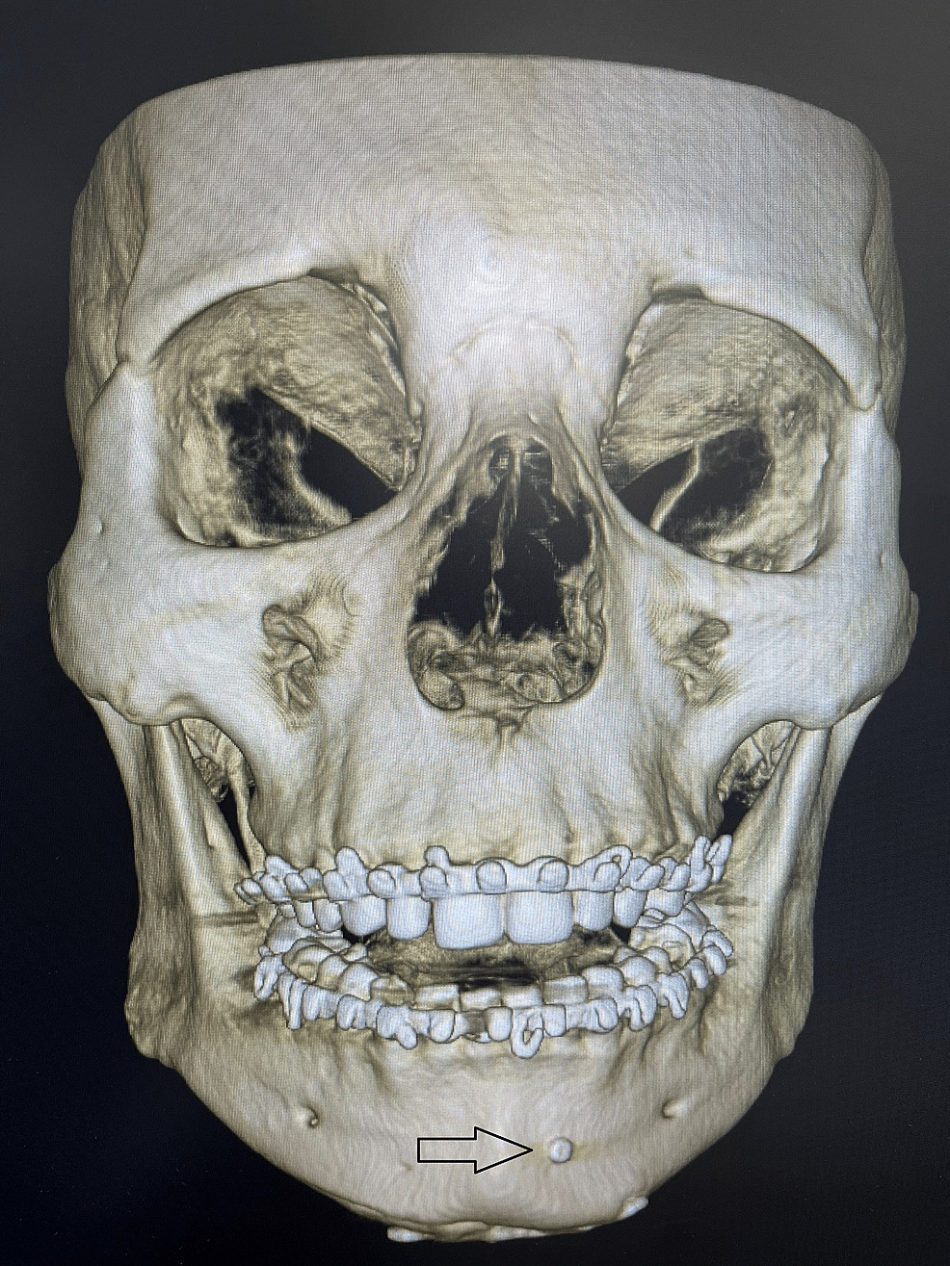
Post-operative radiograph (frontal view) This image shows the postoperative radiograph with 2 x 14mm bicortical screw fixation.
